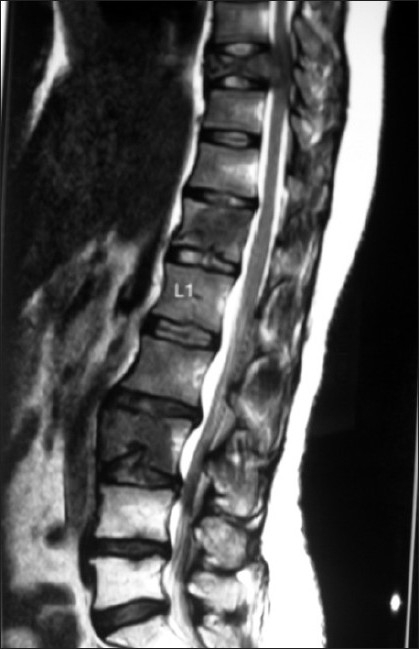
MRI of spine showing the collapse of dorsal 9 vertebra with cord compression
